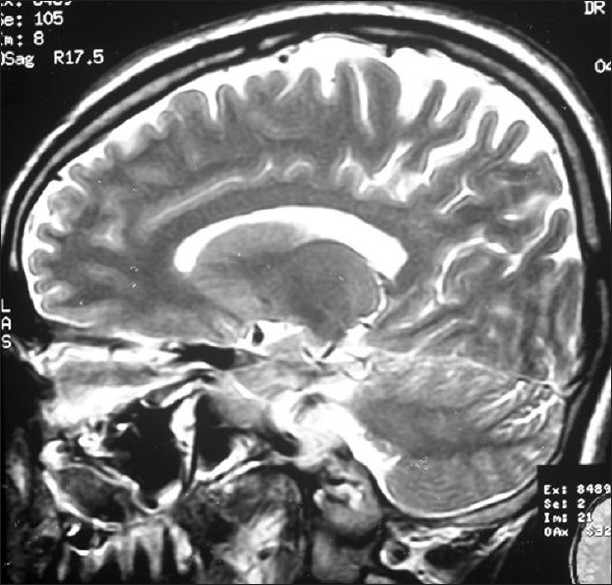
Normal T2 weighted saggital MRI brain in 2003
